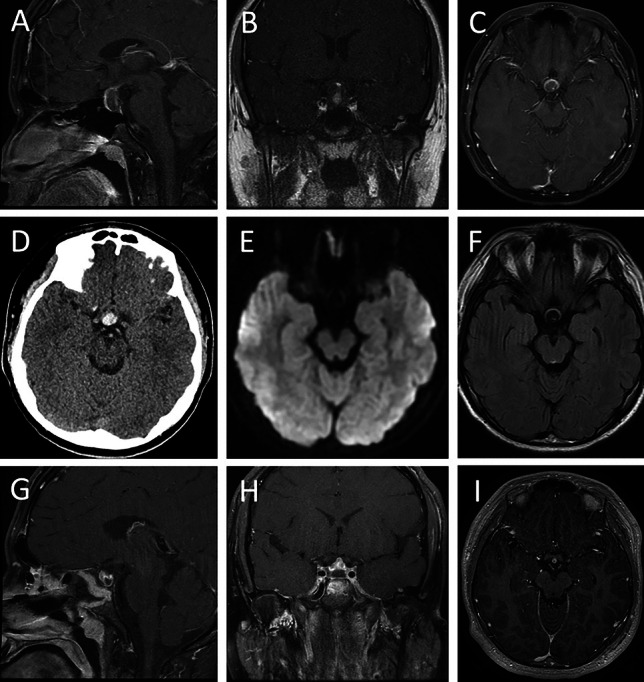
A 14-year-old boy with hemorrhagic RCC. A Sagittal, B coronal, and C axial views of a T1 post-contrast MRI demonstrating a trans-sellar structure measuring 12 × 16 mm with T1 hyperintensity and mildly thickened peripheral enhancement. The lesion abuts the undersurface of the optic chiasm. The stalk enhances normally. The optic chiasm is slightly bowed superiorly. It is hyperdense on CT (D) with Hounsfield units of approximately 70, consistent with hemorrhage. It is not diffusion restricting on DWI (E) and it is hypodense on T2 (F). Postoperative G sagittal, H coronal, and I axial views of a T1 post-contrast MRI show postsurgical changes of transsphenoidal resection within the nasal passages, paranasal sinuses and the sella itself with residual enhancing tissue which likely represents pituitary adenohypophysis
